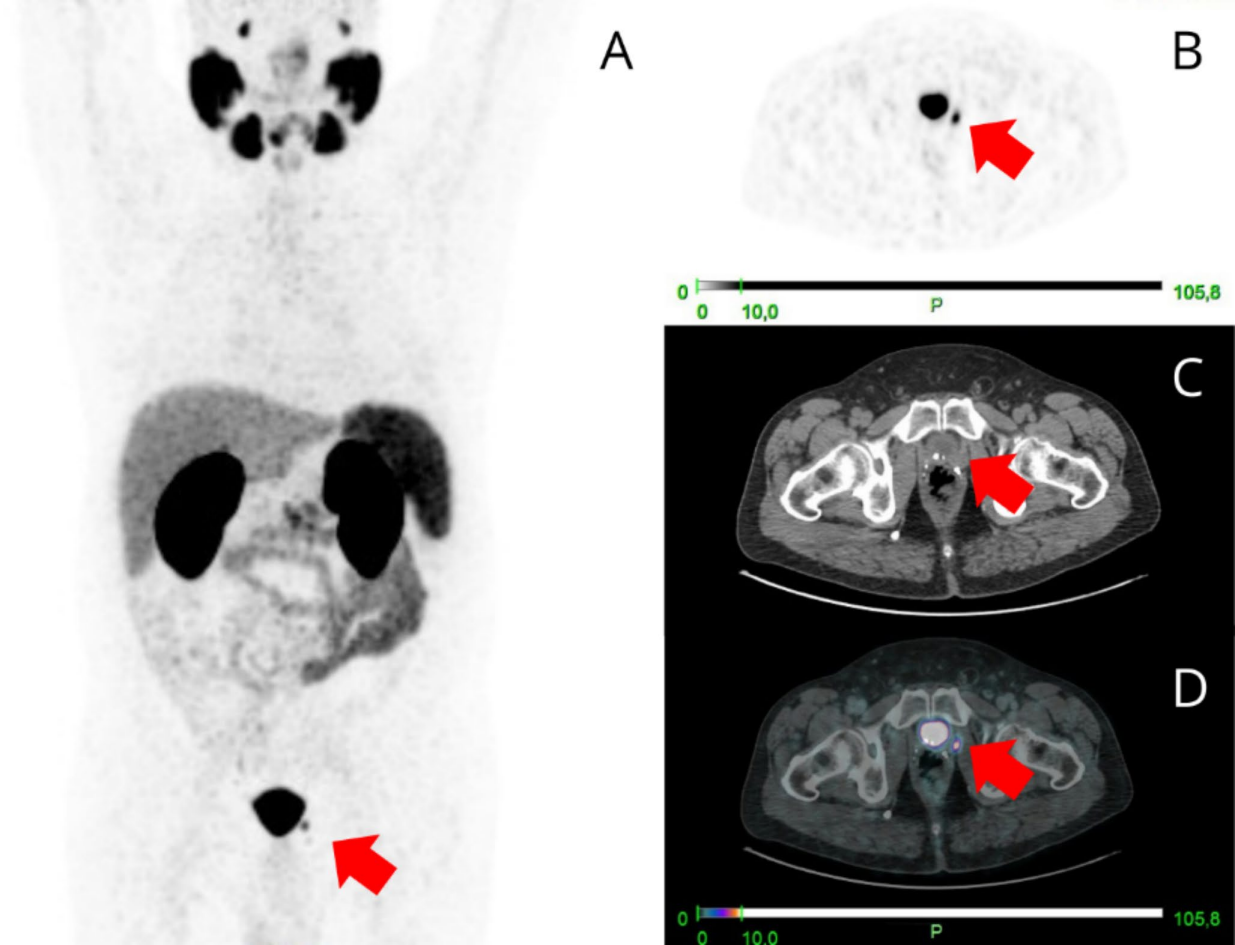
A 68-year-old male with a history of RP for PCa (initial PSA 8.4 ng/mL, Gleason score 4 + 3, ISUP gg 3, PSA post-RP 0.001 ng/mL) experienced a slow PSA rise to 1.37 ng/mL after 71 months, indicating BCR. [⁶⁸Ga]Ga-PSMA PET/CT was performed. Maximum Intensity Projection (A), transaxial PET image (B), transaxial CT image (C), and fused PET/CT image (D) revealed a macroscopic area of intense uptake (SUVmax = 11.6) in the prostate bed (red arrows). sRT was delivered to the prostate bed with concurrent short-term ADT. At 48 months post-sRT, the patient remains with an undetectable PSA
